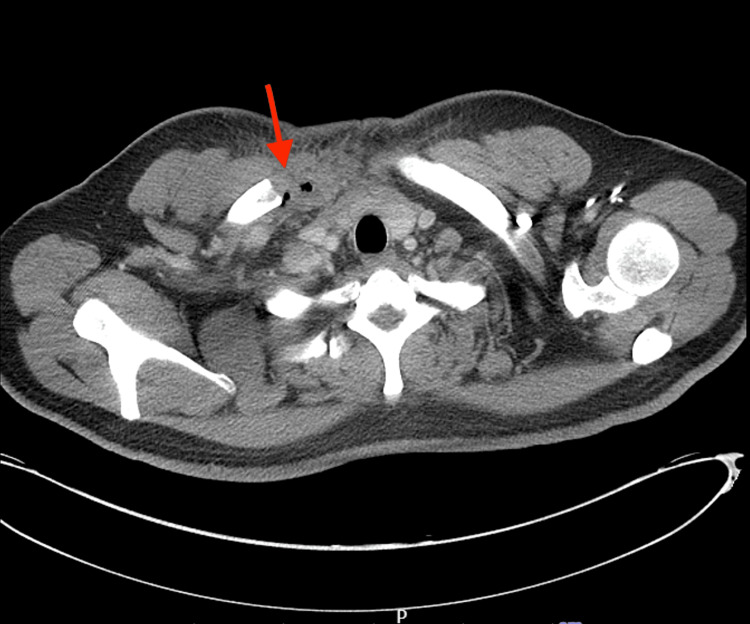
CT scan showing right sternoclavicular joint effusion, associated stranding, bony erosions concerning for septic joint arthritis and osteomyelitis (red arrow)
